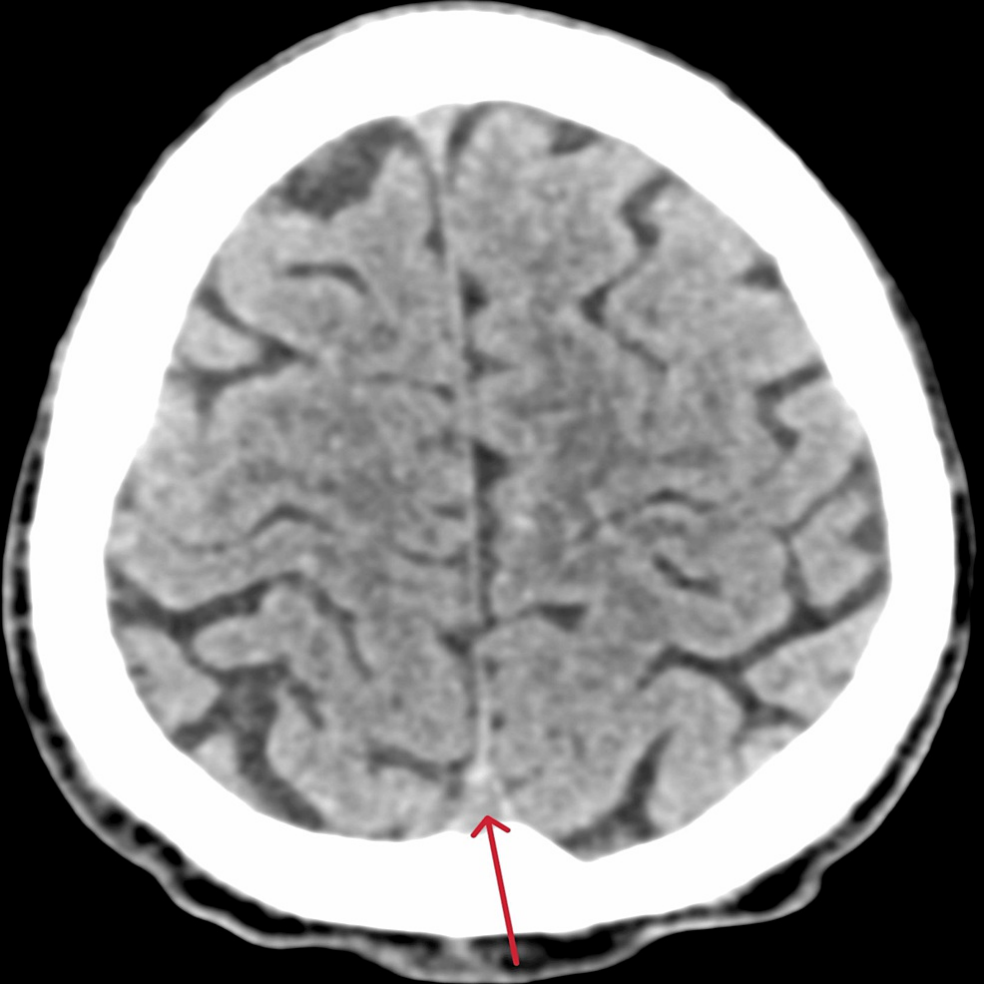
Hounsfield Unit value of non-contrast computed tomography of brain of control patient Arrow showing Hounsfield Unit value of 53.5 in superior sagittal sinus in control patient without thrombosis.
